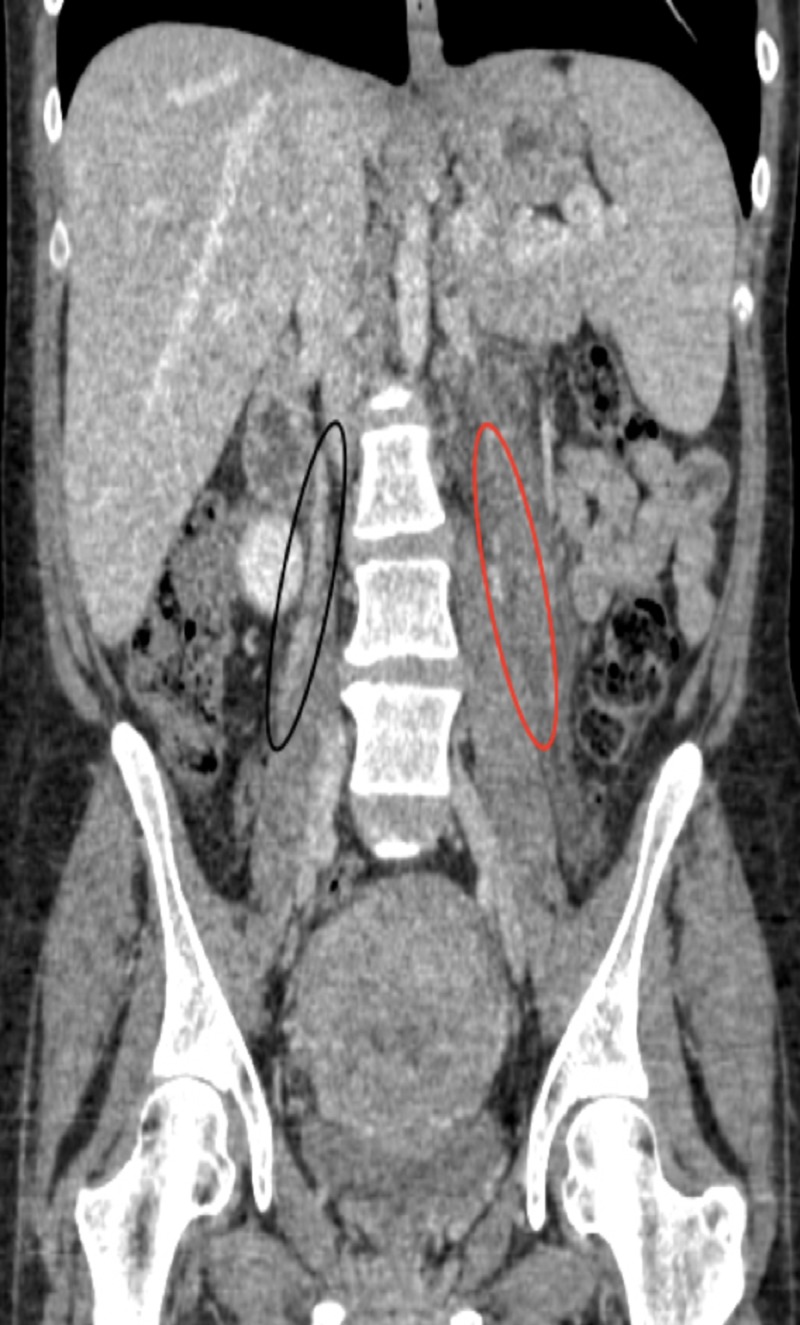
CT scan Coronal contrast-enhanced CT image demonstrates enlargement of Left gonadal/ovarian vein (RED) with central hypo-density and enhancement of vessel wall. Inflammatory changes in adjacent retroperitoneal fat can also be seen. Normal contrast flow through right gonadal vein is shown in (Black).
